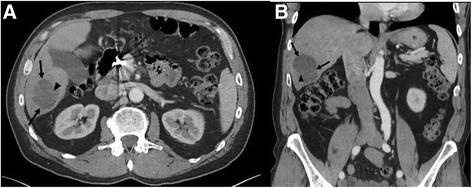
a Axial and b coronal post-contrast CT images through the abdomen approximately 3 months status post Yttrium-90 radioembolization and after the start of evofosfamide clinical trial demonstrate the index metastatic lesion (*arrows*) within the right hepatic lobe with expected post-treatment changes and internal areas of hemorrhage (*arrow heads*), however without a significant decrease in size
